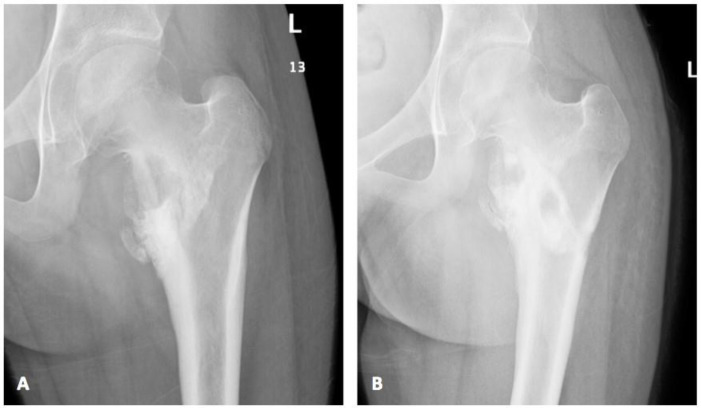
(A) Pre-surgical X-ray showed worsening of medial femoral erosions and destruction with adjacent sclerosis; (B) Post-surgical X-ray demonstrated interval sclerosis and healing with new bone formation along the medial aspect of femoral neck with interval reduction of erosive changes and medial neck lytic lesion.
